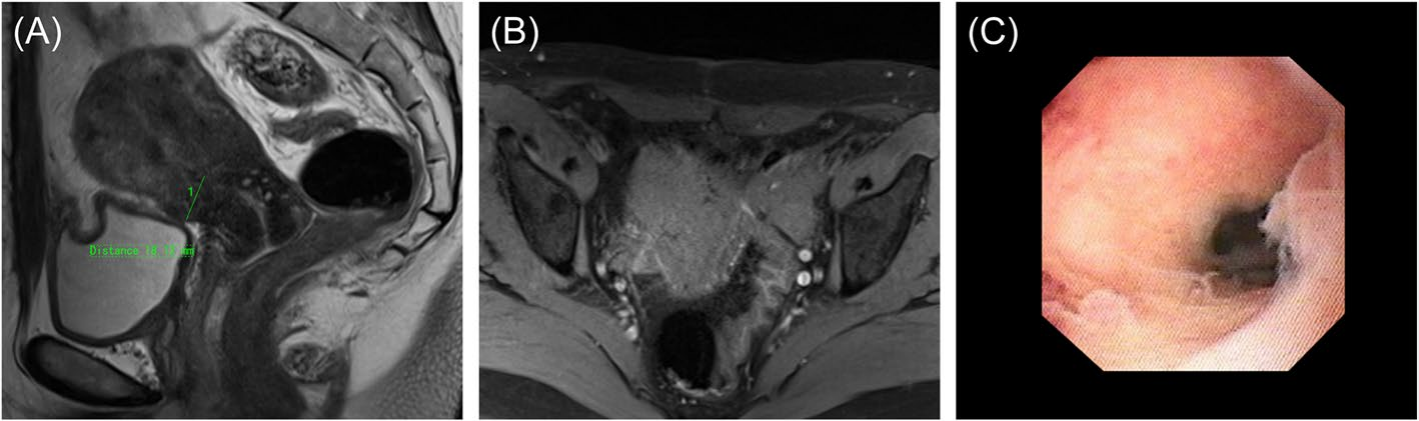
A Sagittal view of a T2-weighted magnetic resonance image showing a residual myometrium thickness of 18.1 mm and the disappearance of fluid retention in the diverticulum after hysteroscopic surgery. B Transverse view of a fat-saturated T1-weighted magnetic resonance image showing the disappearance of high-signal areas suggestive of blood. C Postoperative hysteroscopy revealed neither hypervascularized area nor dendritic vessels
